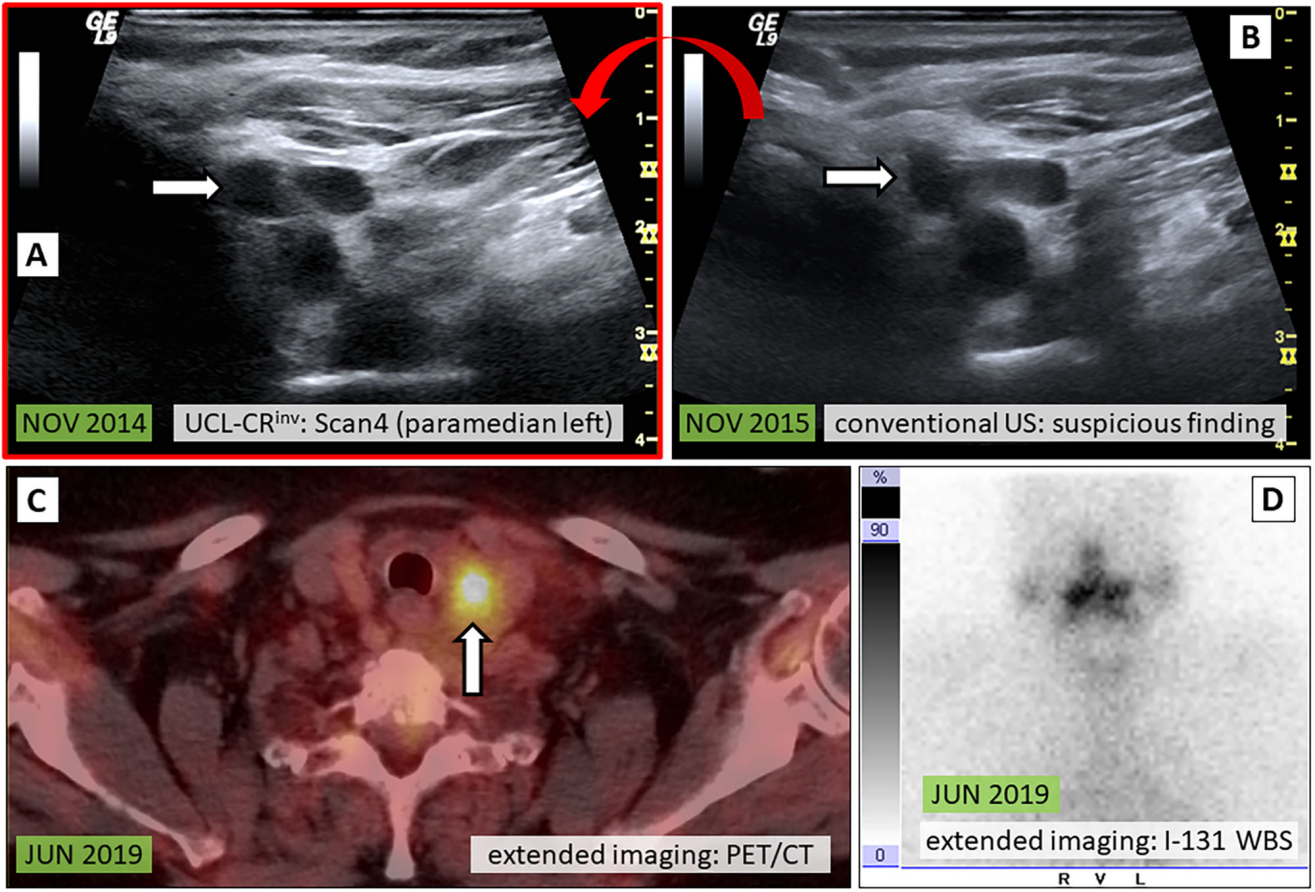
Patient case IV: UCL-CR^inv^ without confirmed diagnosis / false diagnosis. 56-year-old female at initial diagnosis 1999 (PTC pT2 cN0 cM0). Conventional US revealed a suspicious nodule in the former left-sided thyroid lodge, measuring 12 mm [B, white arrow] after 6 years (NOV 2015). The retrospective PACS review of the UCL from NOV 2014 revealed the same lesion without significant increase in size [A, white arrow], which was consequently assessed as benign (scar). However, TG levels were slowly rising throughout the following years and finally additional extended imaging was conducted. A pathological FDG uptake was seen on F-18-FDG PET/CT [C, white arrow], but I-131 WBS did not show iodine uptake [D, white arrow]. Resection of the lesion confirmed a local tumor relapse of the PTC. UCL-CR^inv^ standardized cervical ultrasound cine loops with clinical relevance - invalidation of a suspicious finding identified on conventional live US, PTC papillary thyroid carcinoma, US ultrasound, PACS Picture Archiving and Communication System, TG thyroglobulin, F-18-FDG PET/CT fluorine-18-fluorodeoxyglucose positron emission tomography computed tomography, I-131 WBS iodine-131 whole body scintigraphy
